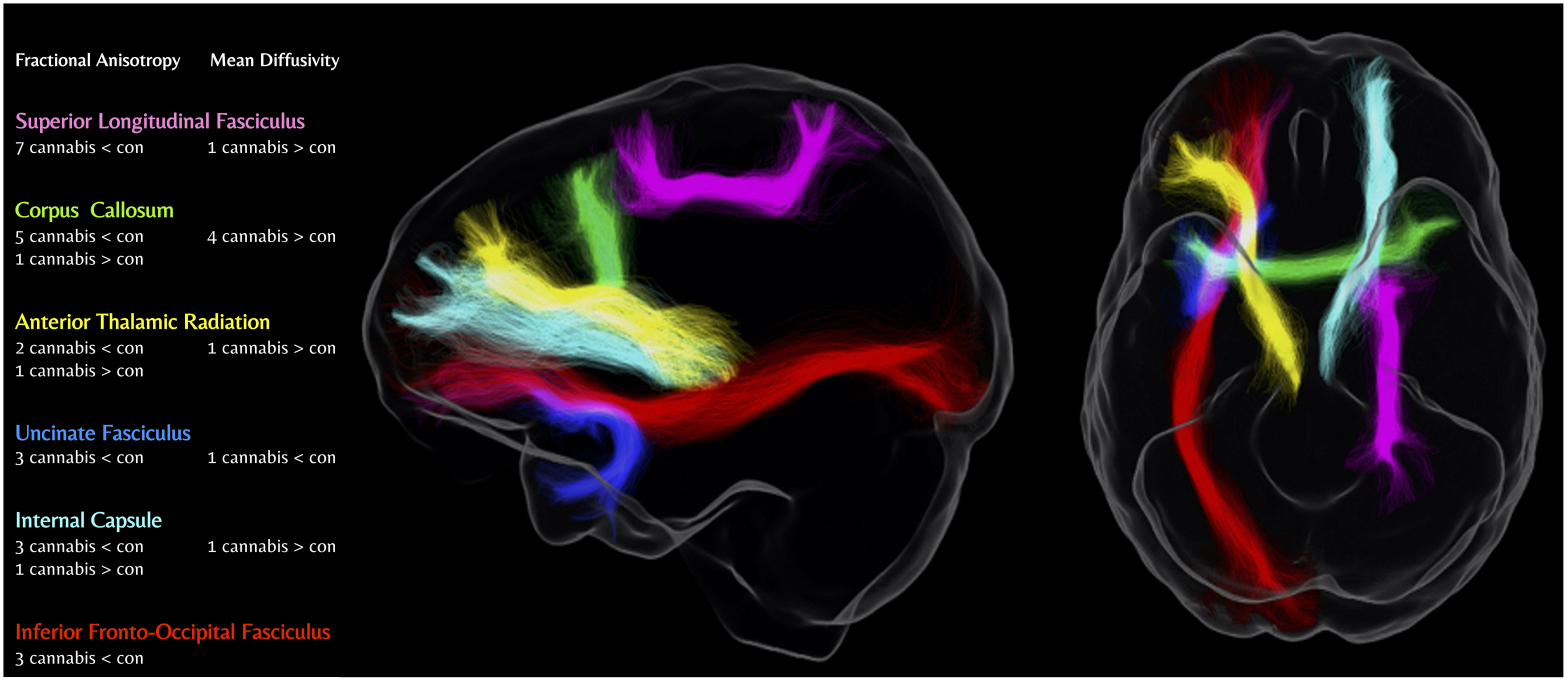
Number of diffusion-weighted MRI studies showing differences in major white matter tracts in cannabis users compared to controls. Cannabis < Con; Lower metric of white matter microstructure in cannabis users vs. controls, Cannabis > Con; Higher metric of white matter microstructure in cannabis users vs. controls. Figure produced using MRtrix3 and tracts generated from TractSeg (Wasserthal et al., 2018; Tournier et al., 2019).
